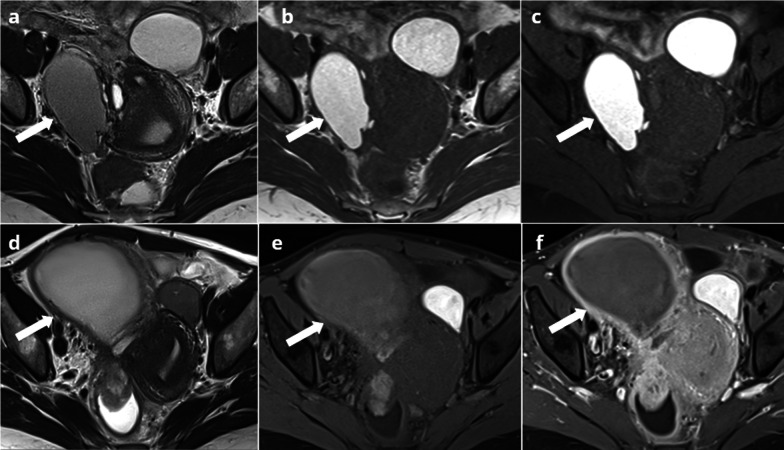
39-year-old female with deep endometriosis. Typical endometrioma of the right ovary (arrow): unilocular cyst with shading on T2W images (a) hypersignal > fat on T1W images (b), persisting on T1W FS images (c). Several months later, she presents with pelvic pain, fever and hyperleukocytosis. MRI shows an increase in size of the right endometrioma (arrow), with loss of shading on T2W (d), loss of hyperintensity on T1W FS (e) and a thick, enhanced wall on T1W FS after contrast injection (f)
